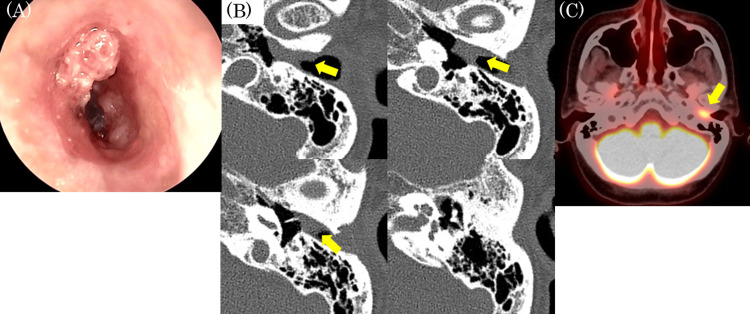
Findings at the time of re-referral (A) Endoscopic findings in the left external auditory canal: Papillary lesions were found around the external auditory canal and on the posterior half of the tympanic membrane. (B) CT (axial): The soft tissue shadow was localized within the left auditory canal and there was no obvious bone destruction. (C) PET-CT: Abnormal accumulation was found only in the left auditory canal.
